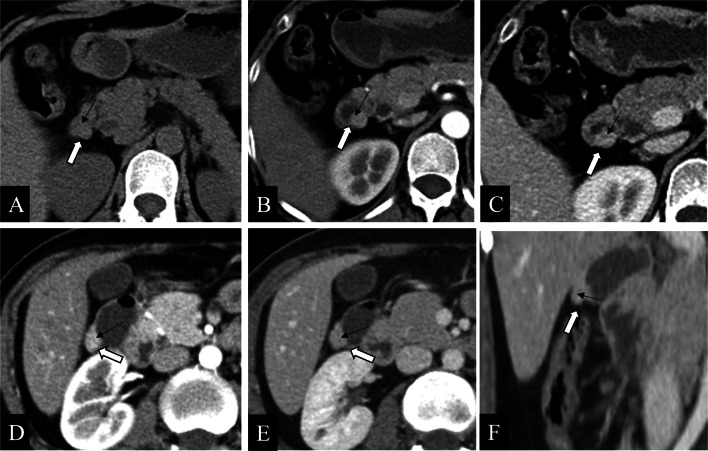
a–c Axial plain (a) /arterial (b) /venous (c) phases CT images of a heterotopic pancreas in the duodenum of a 57-year-old female showed a 1.2 cm, round, well-defined, and mixed-growing lesion (white arrows). Small duct-like structure (black arrows) was vaguely seen within the lesion in each phase, presenting slightly hypo density compared to the surroundings. d–f Axial arterial (d) /Venous (e) /coronal venous (f) phases CT images of a heterotopic pancreas in the duodenum of a 58-year-old male showed a 1.3 cm × 0.9 cm, flat lesion (white arrows) with a lobulated contour and an exophytic growth pattern. Small duct-like structure (black arrows) was seen within the lesion in each phase
